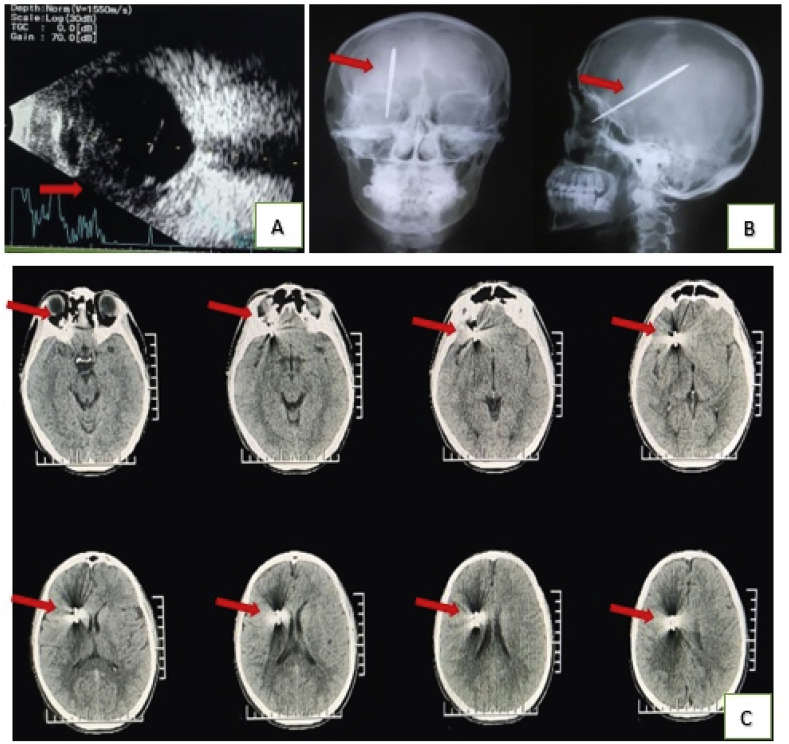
Radiological Findings: A). Ocular ultrasonography presenting a discontinuity of the inferior wall of the eyeball (arrows). B). Plain skull x-ray (anteroposterior and lateral view) showing the homemade metal arrow penetrating the right orbital roof and traversing to the ipsilateral parietal lobe (arrows). C). Non-contrast CT of the brain depicting the metal arrow's trajectory posteriorly through the right orbital roof (arrows).
